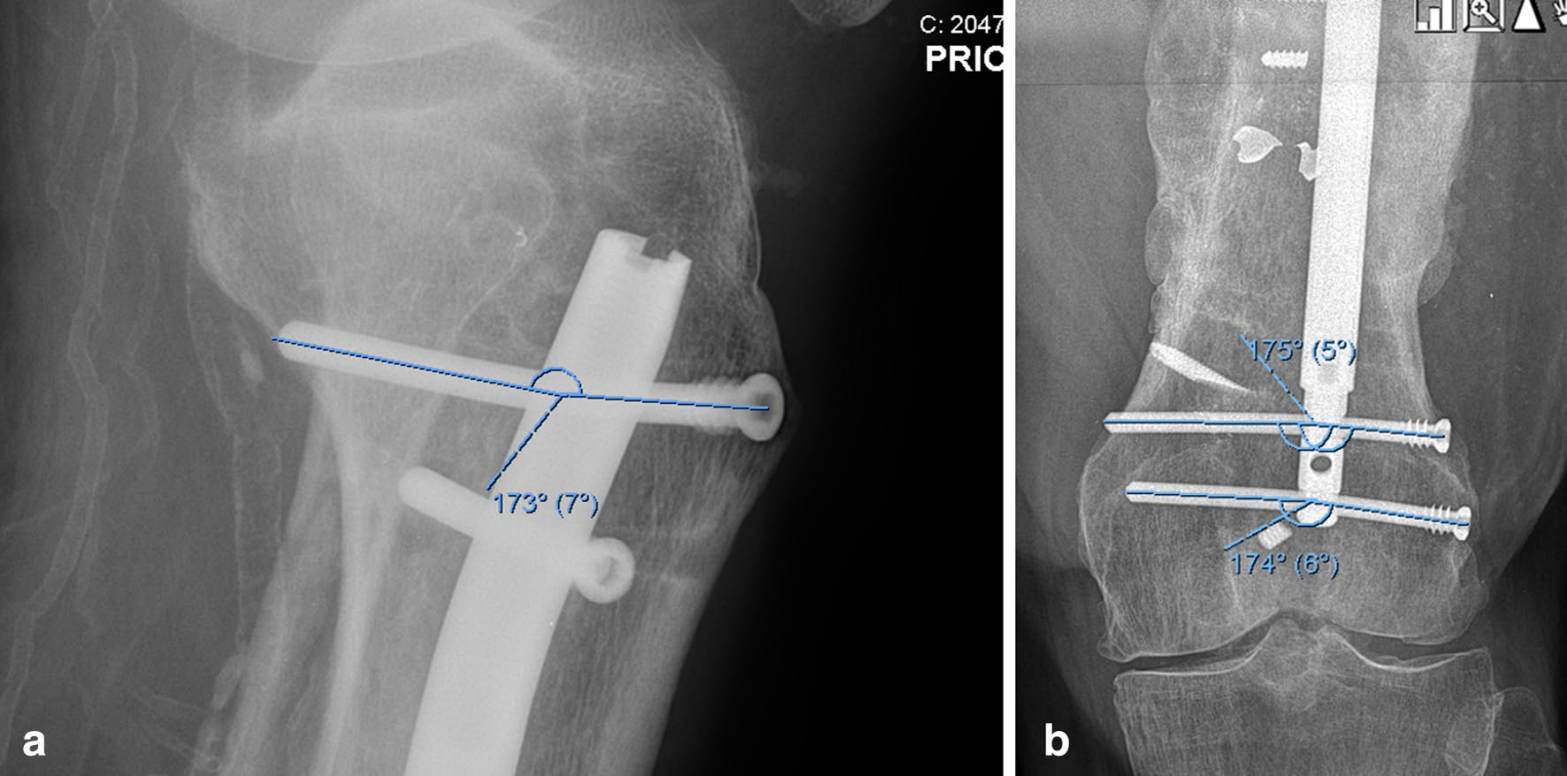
a The amount of screw bending can be quantified using an angular measurement. In this case the proximal locking bolt is bending 7°. b In this radiograph the bolts are bending 5° and 6°
